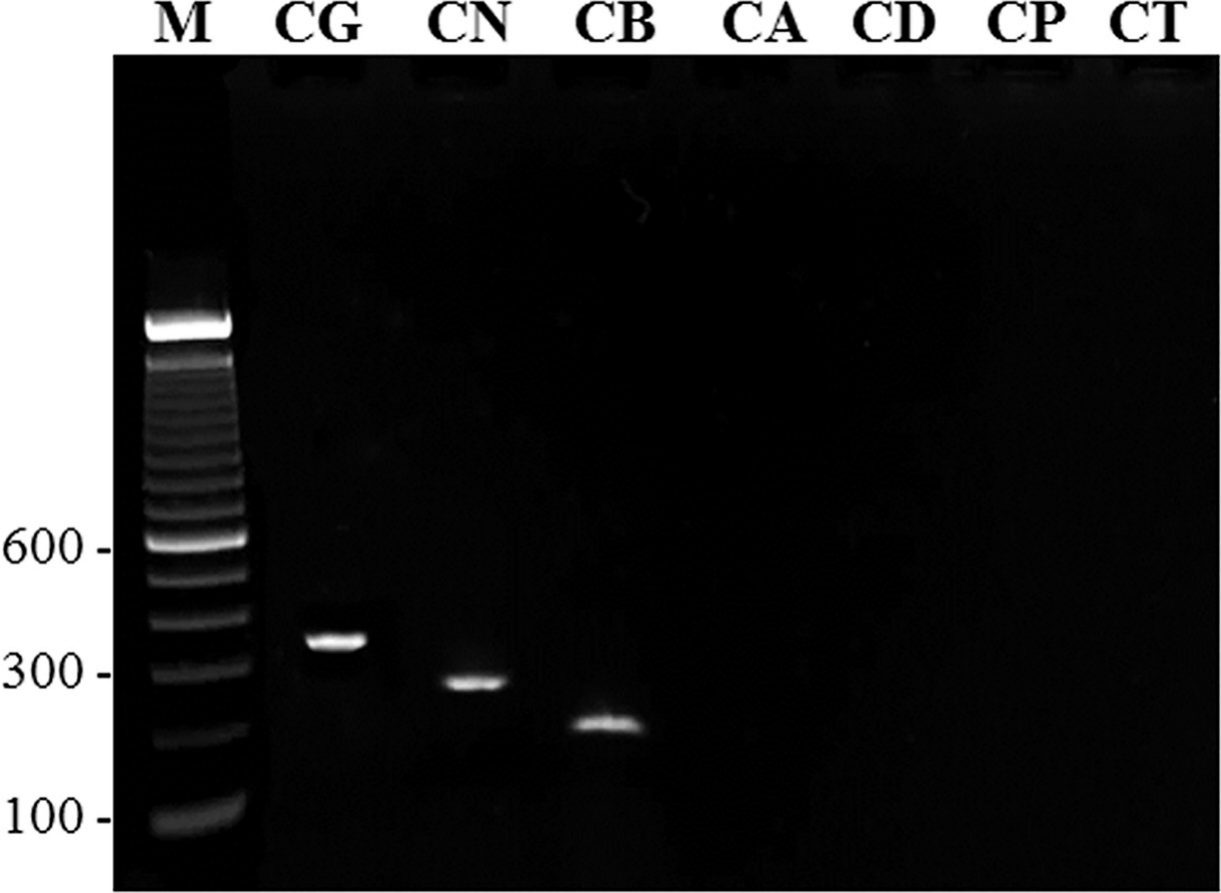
An agarose gel of amplified products obtained in mPCR using *C*. *glabrata* sensu stricto-specific (mCGLF), *C*. *nivariensis*-specific (mCNIF) and *C*. *bracarensis*-specific (mCBRF) forward and *C*. *glabrata* complex-specific (mCGCR) reverse primer with genomic DNA from reference strain of *C*. *glabrata* sensu stricto ATCC 90030 (lane CG), *C*. *nivariensis* CBS 9983 (lane CN), *C*. *bracarensis* CBS 10154 (lane CB), *C*. *albicans* ATCC 76615 (lane CA), *C*. *dubliniensis* CBS 7987 (lane CD), C. *parapsilosis* ATCC 22019 (lane CP) and *C*. *tropicalis* ATCC750 (lane CT). Lane M is 100 bp DNA ladder and the position of migration of 100 bp, 300 bp and 600 bp fragments are marked.
